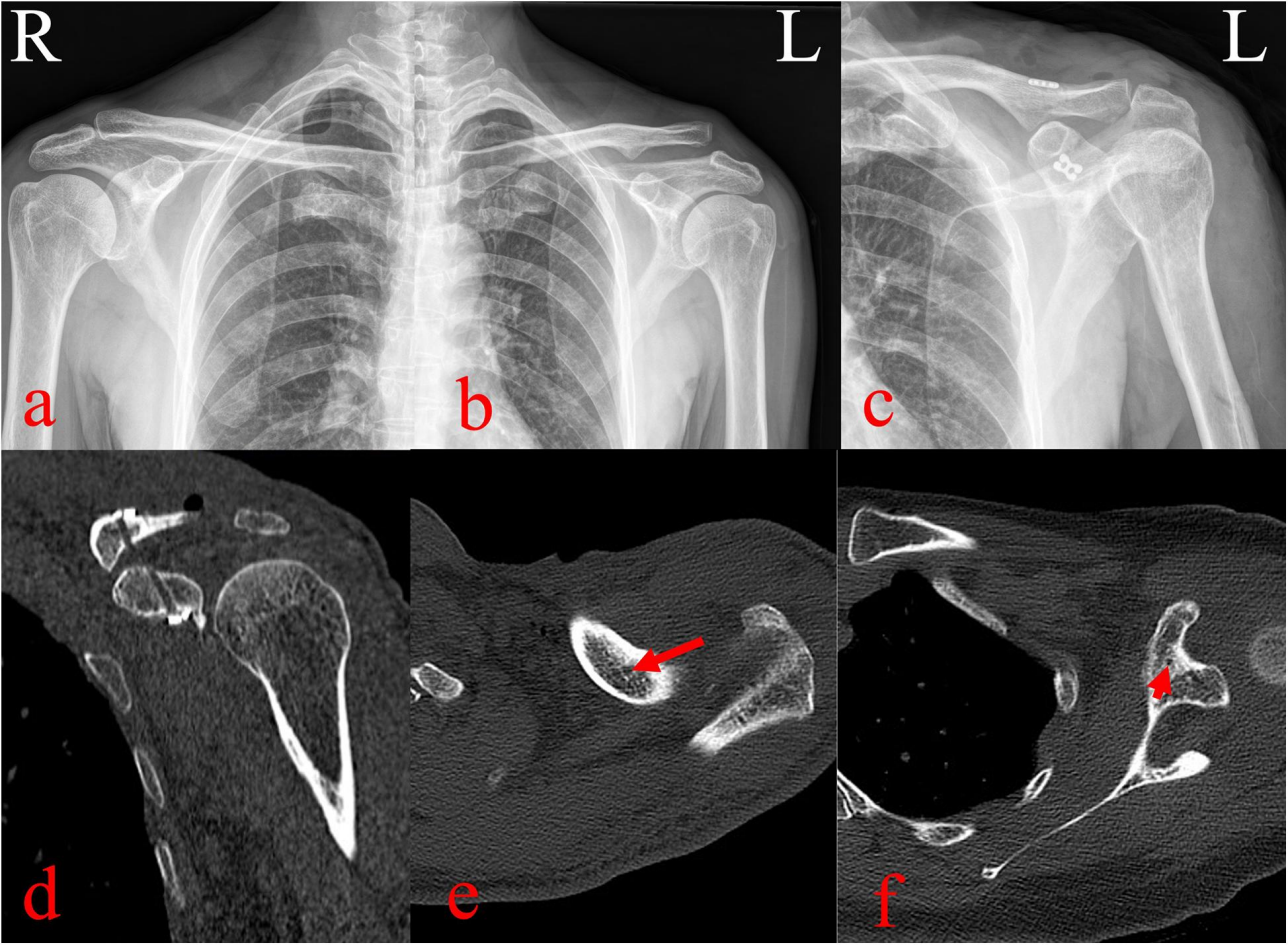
Pre- and postoperative imaging evaluation. (a–b) Preoperative radiographs showing >100% displacement of the left AC joint. (c) Postoperative radiographs showing anatomic reduction with appropriate placement of the ALP and four-leaf clover plate. (d–f) Three-dimensional computed tomography reconstructions confirming central positioning of clavicular and coracoid bone tunnels with satisfactory implant placement.
